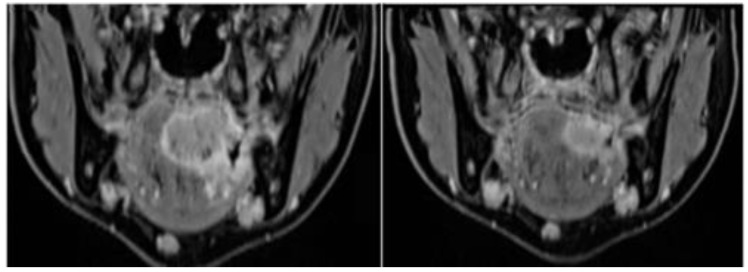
Contrast-enhanced MRI demonstrating a large, heterogeneous squamous cell carcinoma of the left tongue base, with irregular enhancement and infiltration of adjacent soft tissues, arising 14 years after IMRT for Epstein–Barr virus–associated nasopharyngeal carcinoma. The lesion is located entirely within the previously irradiated field in a patient without additional known risk factors.
